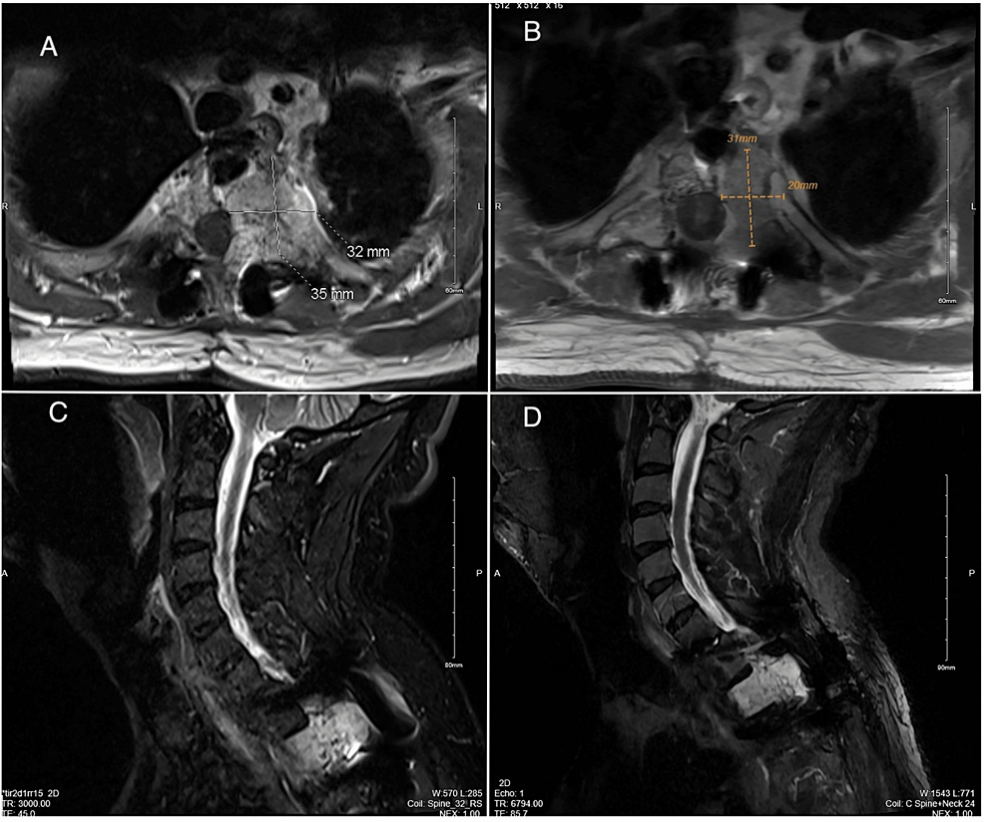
Post contrast T1 weighted pre (A, C) and post (B, D) hypofractionated SBRT MRI images of thoracic spine showing interval disease regression.
